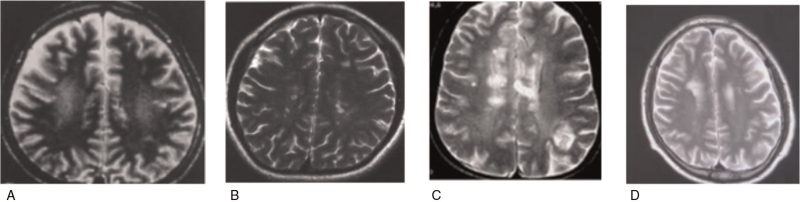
The cranial Magnetic resonance imaging (MRI) results.
